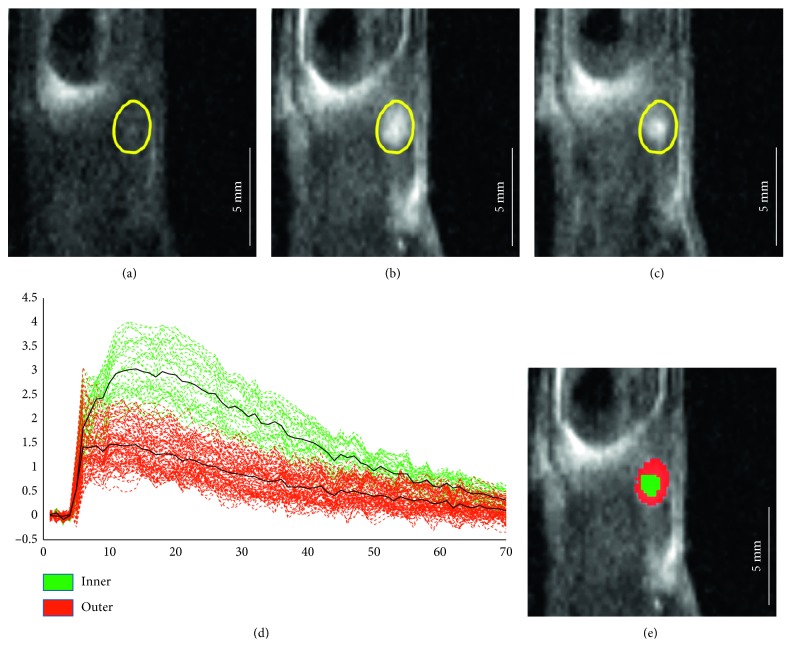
Precontrast (a), early (b), and late (c) DCE-MRI images (the same slice reported in Figures 1(a)–1(c) is shown) of a representative lymph node (#15). The region of interest (in yellow in (a)–(c)) was manually drawn to encompass the whole lymph node. The long-axis size of this lymph node was around 2.5 mm. k-means clustering assigned each pixel-based NDE curve (thin dashed lines) to one of the two clusters (d); cluster-averaged NDE curves are also shown (thick black continuous lines). The obtained segmentation map is shown with the corresponding colours (e).
